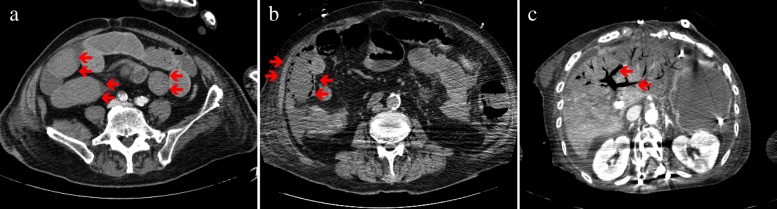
Red arrows indicate the CT findings of the diagnosis of NOMI in each images: a absence of bowel wall enhancement, b pneumatosis intestinalis, and c portal venous gas
